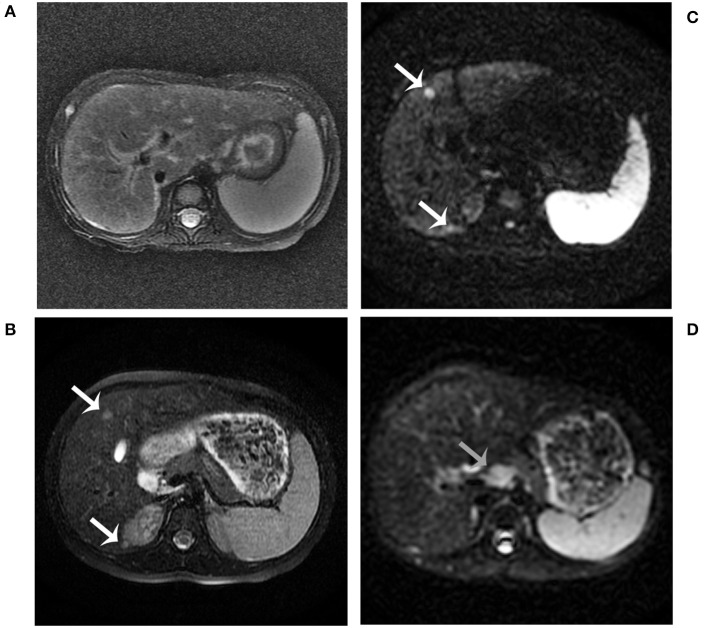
Abdominal MRI findings. MRI examination was performed in the patient at age 2 years. It showed hepatosplenomegaly, portal tract edema, and a slightly low signal intensity of the liver on T2-weighted imaging (A). MRI combined with diffusion-weighted imaging (DWI) examination was performed in the patient at age 5 years (B–D). The nodules showed a slightly high signal intensity on T2-weighted imaging (B, white arrows). The nodules showed restricted diffusion on DWI (C, white arrows). Enlarged retroperitoneal lymph nodes showed restricted diffusion on DWI (D, gray arrows).
